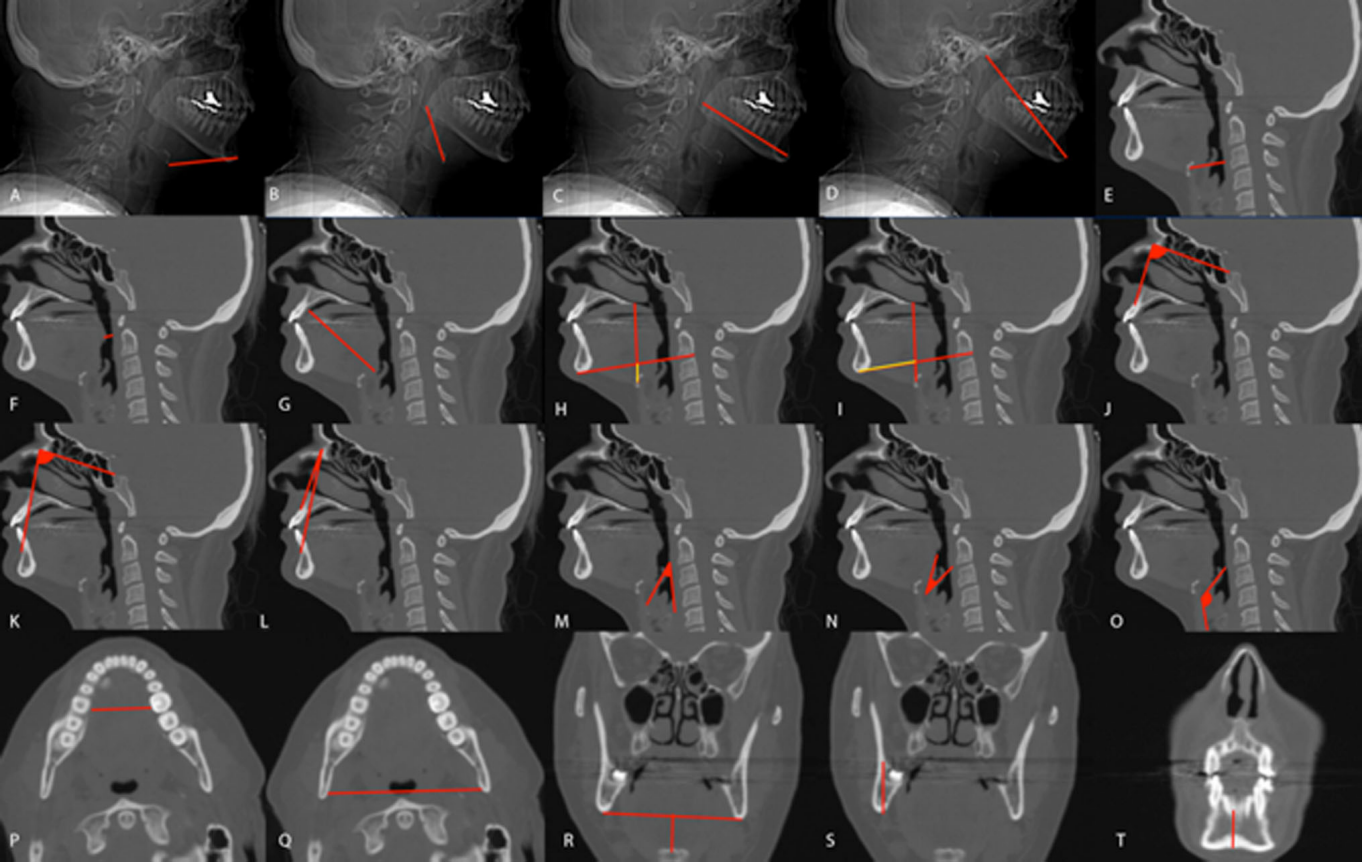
Various cephalometric measurements obtained from preoperative imaging. These measurements include: a hyoid to gnathion, b hyoid to gonion, c gonion to gnathion, d condyle to gnathion, e posterior pharyngeal wall to hyoid, f posterior pharyngeal wall to soft palate, g tip of tongue to vallecula, h, i two intersecting lines were created in each radiograph, one line from the gonion and to the base of vertebral body of C2, and another line from the posterior aspect of the hard palate and to the hyoid. The distance from the intersection of these two lines to the gonion and hyoid were subsequently recorded and evaluated independently and as a ratio. Angles measurements included, j–l measured involving the sella, nasion anterior nasal spine and suprametale, m measured between the epiglottis and posterior pharyngeal wall, n epiglottis and BOT and o epiglottis and larynx. Mandibular measurements included, p, q intermandubular distance at two points, r distance between the lower edge of the mandible and the hyoid, s, t anterior and posterior mandibular height
